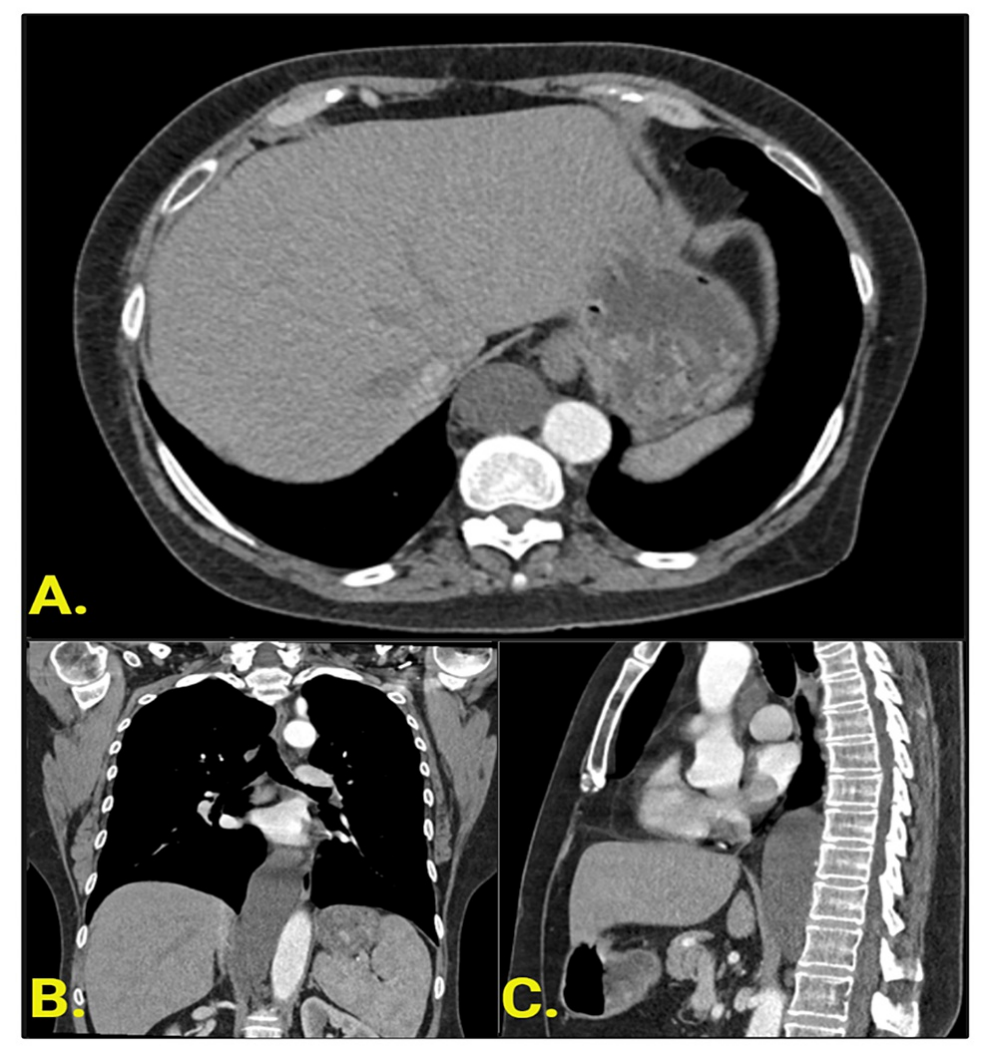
CT Chest with IV Contrast (A) Axial section demonstrating a round, well-marginated, right retrocrural lesion with homogenous attenuation (mean 13.3 HU) without enhancement and no apparent invasion of adjacent structures. (B) Coronal and (C) sagittal reformats of the same lesion demonstrate its tubular structure extending from the level of the T12 vertebral level inferiorly to the T8 vertebral level superiorly.
